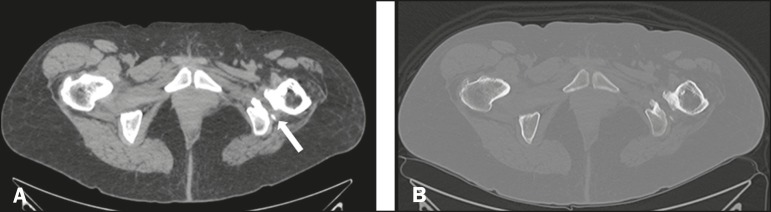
A: Computed tomography slice in the axial plane, with a soft tissue
